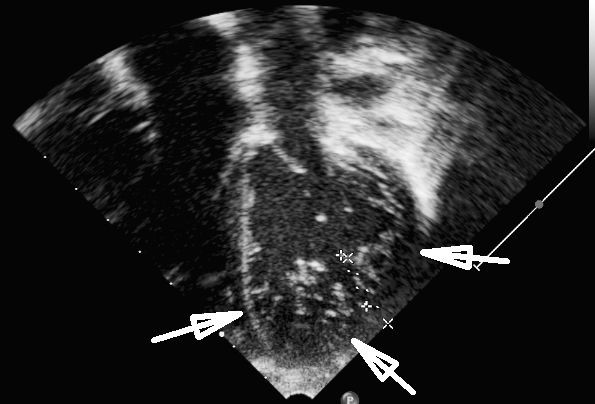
Echocardiographic apical 4-chamber-view showing the hypertrabeculated left ventricular apex with a two-layered structure.
